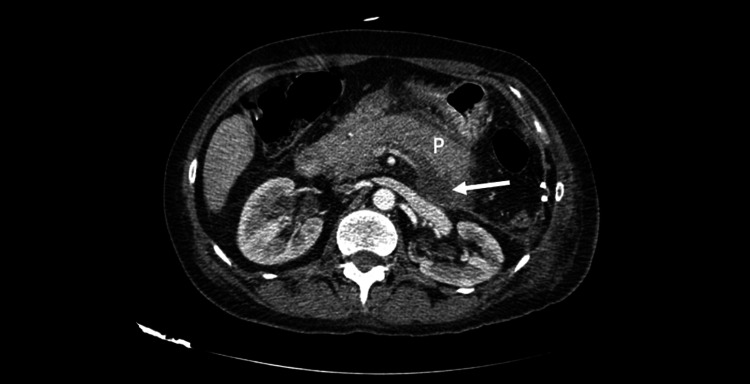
Abdominal computed tomography with contrast showing acute pancreatitis with diffuse swelling of the pancreas (P) and retropancreatic fluid collection with no signs of necrosis (arrow).
